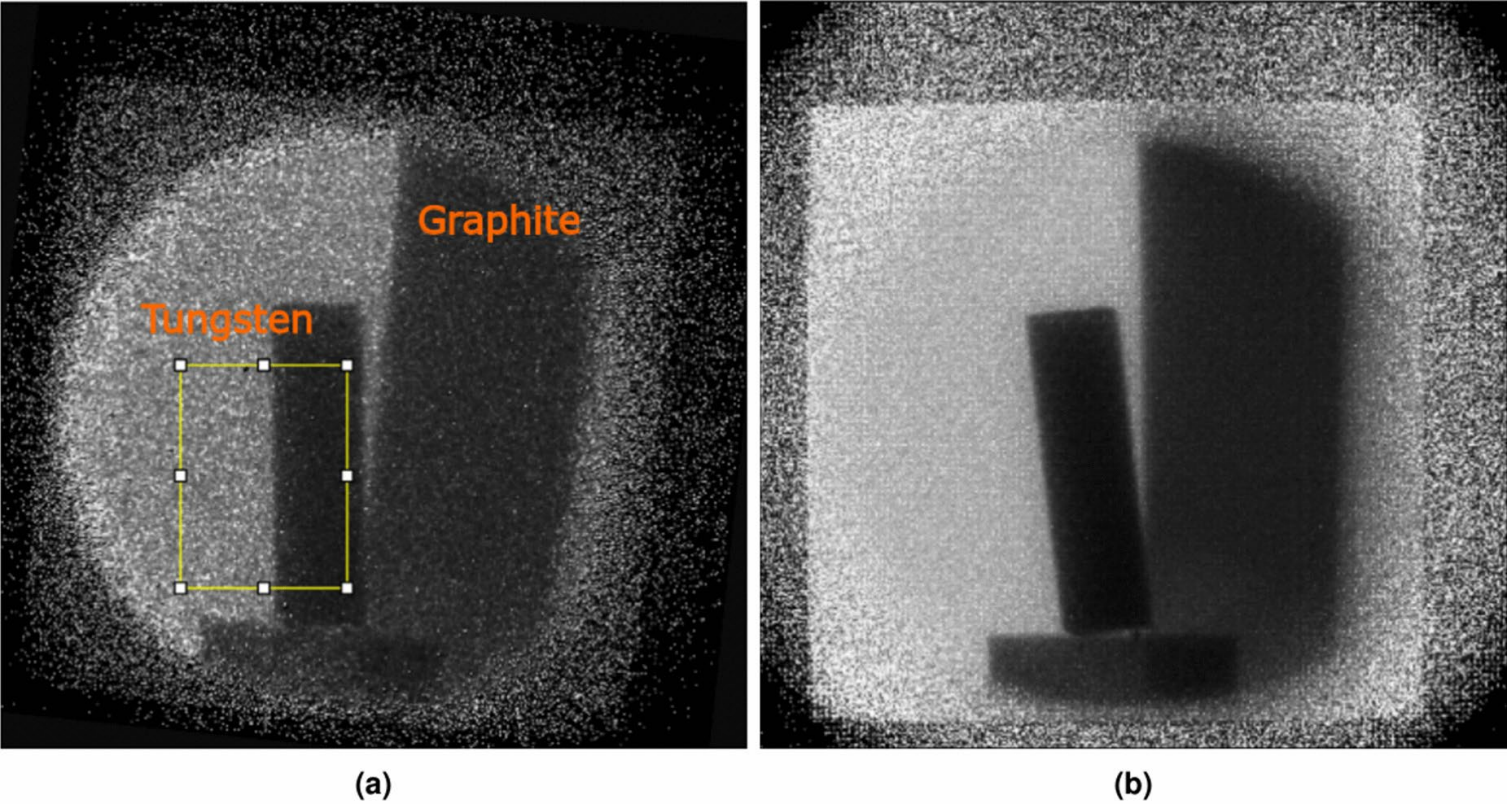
Radiograph of a tungsten and a graphite block used for the parameter scan (neutron energies 2.5 MeV to 8 MeV). Data was evaluated with the highest resolution parameter set (a) and the highest contrast parameter set (b). (a) The yellow box shows the region-of-interest for which the line-spread function and the pixel intensity histogram were determined. The image was rotated clockwise $$6^{\circ }$$. (b) Unlike (a) the image has not been rotated.
